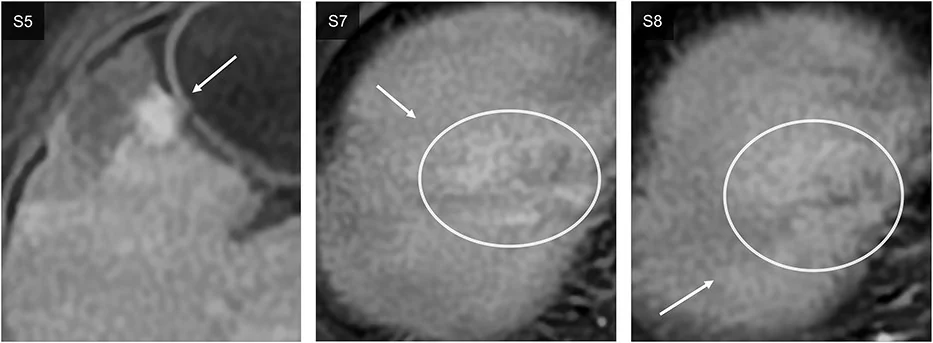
EOB-MRI at 3 months following conventional transarterial chemoembolization, in the arterial phase. Residual arterial enhancement persists in the treated segment 5 lesion, while non-target lesions in segments 7 and 8 show no clear improvement.
